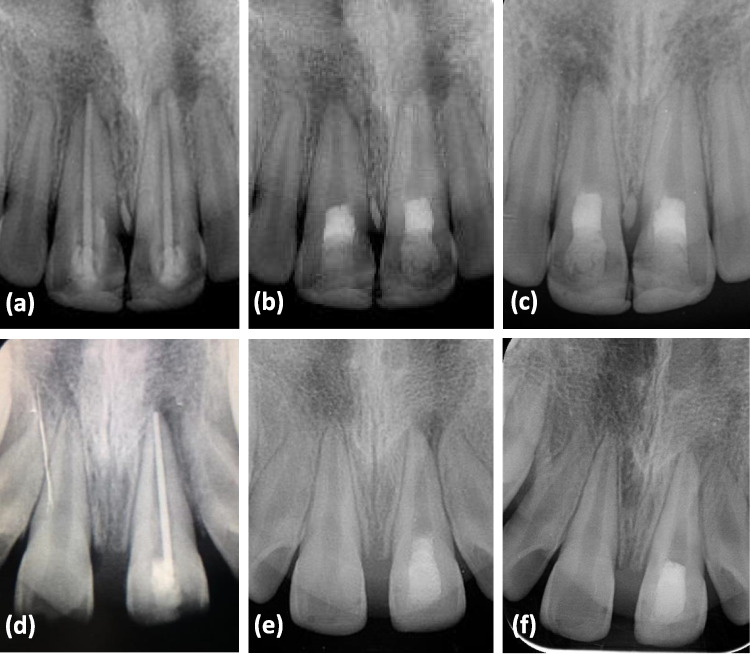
Periapical radiographs of two patients retreated with platelet-rich fibrin (PRF) (a, b, and c) and revascularization with induced blood clot formation (BC) (d, e, and f). a Preoperative radiograph of a 14-year-old male with periapical radiolucency related to root canal retreatment on maxillary central incisors; b and c continuous reduction in size of periapical radiolucency after 6 months and 12 months of follow-up; a preoperative radiograph of a 13-year-old female with periapical radiolucency related to root canal retreatment on a right maxillary central incisor; b and c continuous reduction in size of periapical radiolucency after 6 months and 12 months of follow-up
